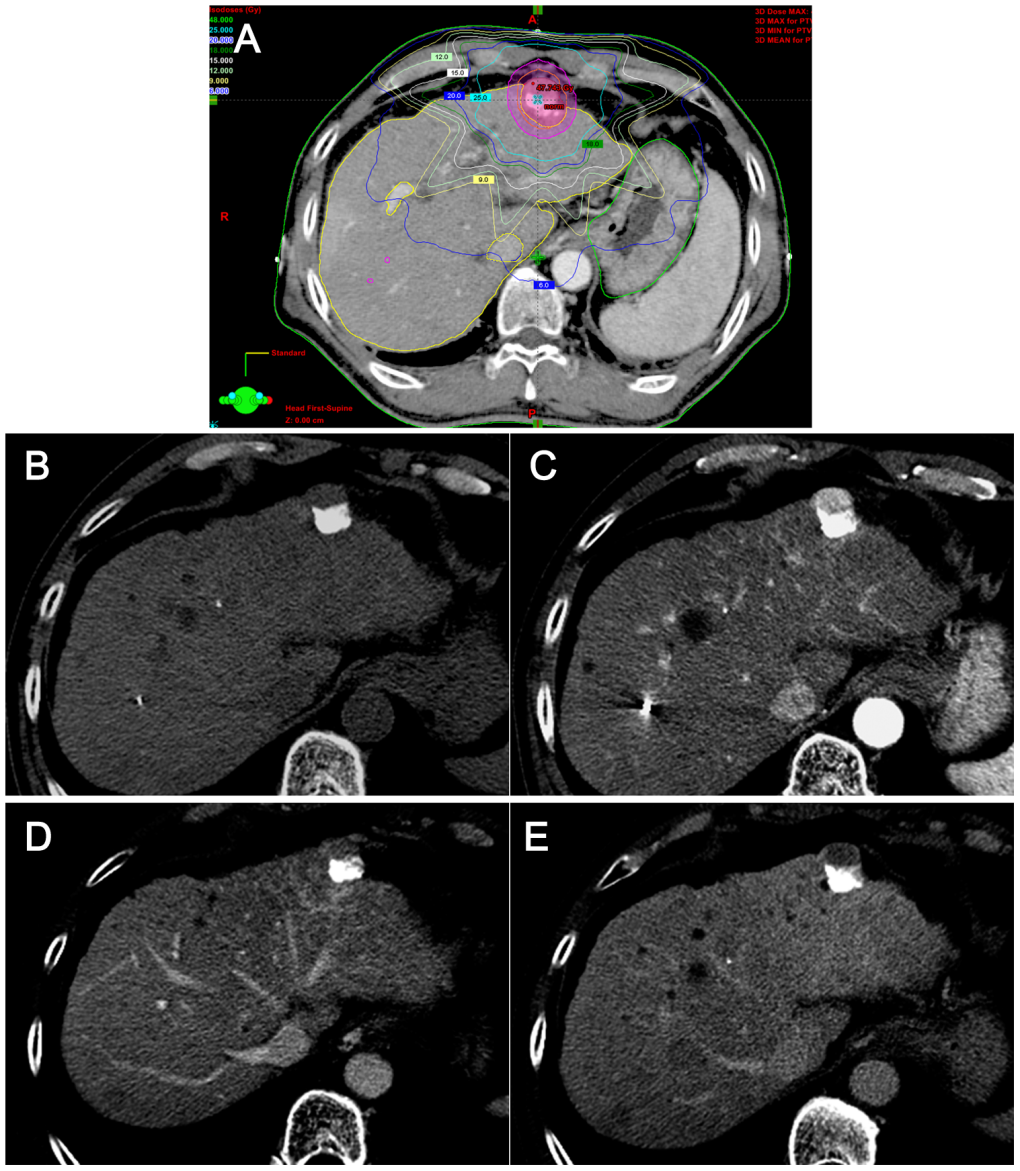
Typical hepatic parenchymal change seen on one month after SBRT. (A) As seen on the isodose curve for planning SBRT with a total dose of 45 Gy, the planning target volume (PTV, purple circle) is expanded to cover the gross tumor volume (red circle). The irradiated hepatic parenchyma is delineated corresponding to the region inside the 15-Gy isodose line, but outside of the PTV boundary. (B-E) The target tumor (arrowheads) in the left lateral segment of the liver, was treated with transarterial chemoembolization prior to SBRT. Residual tumor is noted (arrowheads) with arterial enhancement and washout on the portal and delayed phases adjacent to lipiodol. One-month f/u CT images after SBRT demonstrate that there is no significant change in the irradiated hepatic parenchyma or in the tumor (arrowheads) in any phase including noncontrast (B), arterial (C), portal (D), and delayed (E) phases. A fiducial marker (arrows) is also seen.
